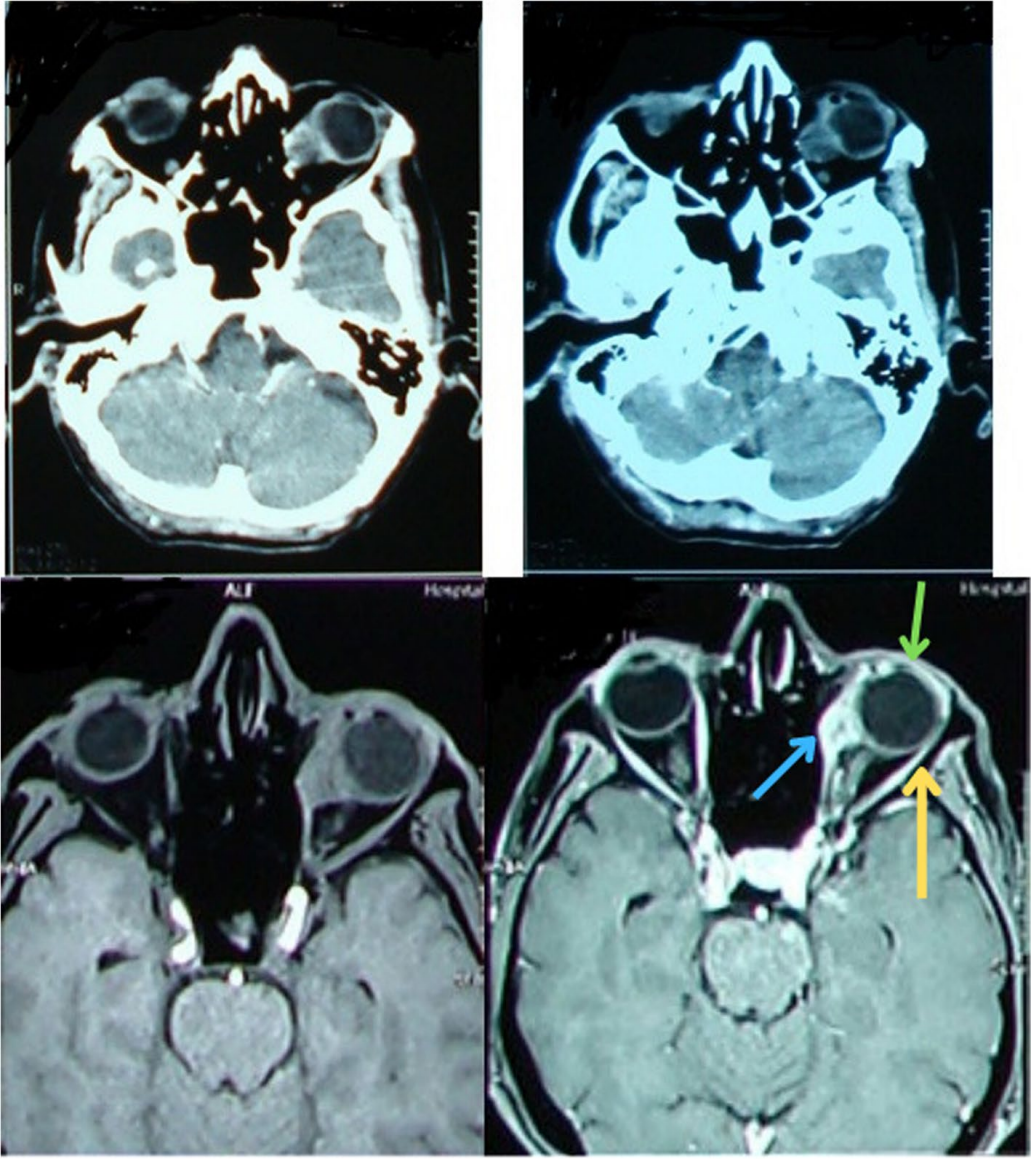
CT scan & MRI of patient revealing left intraconal mass before and after operation (Top right-CT scan before operation. Top left-CT scan after operation. Bottom right-T1 MRI before operation (Note the Lateral recuts: yellow arrow, Medial recuts and tumor compressing the globe: blue arrow, globe: green arrow). Bottom left-T2 MRI before operation). Because of the diagnostic nature of the operation the size of the tumor is not changed significantly
